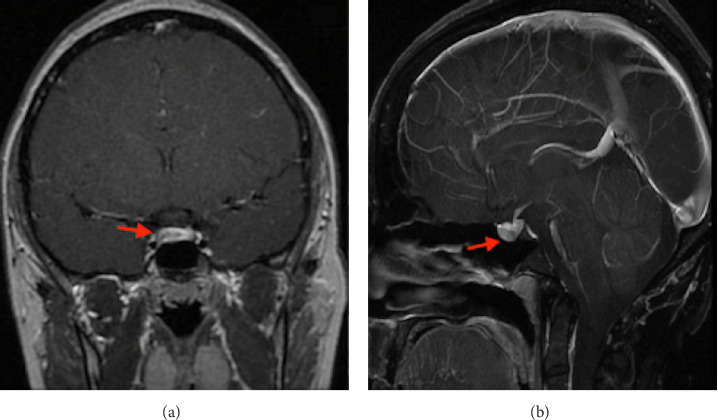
Magnetic resonance imaging, T1 post-gadolinium. (A) Coronal view and (B) Sagittal view. A 3 × 4.6 × 3.7 mm microadenoma is observed (red arrow).
